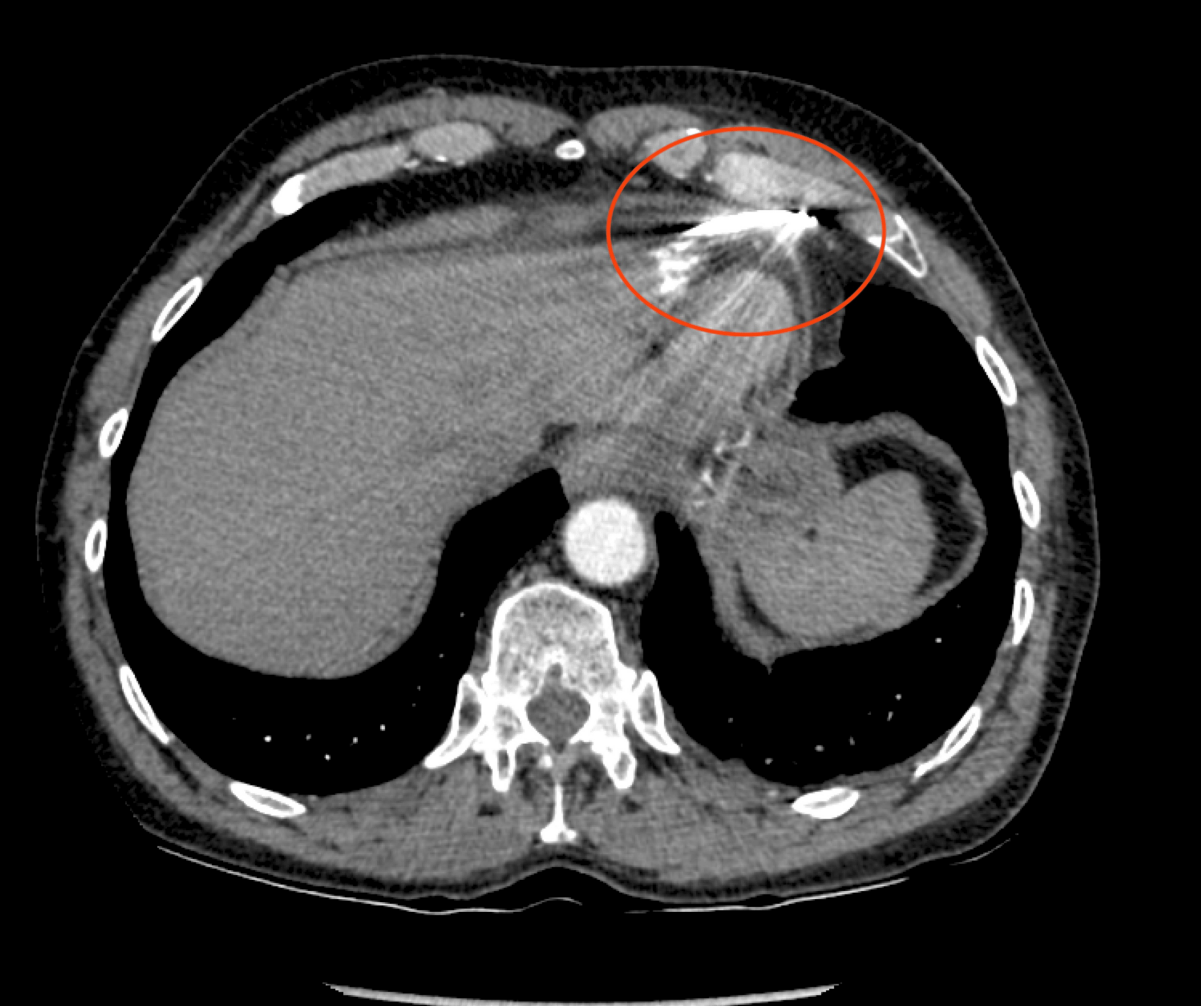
Pacemaker Lead Perforating Right Ventricle (RV) Found on Image Review 2-D computed tomography demonstrating RV lead perforation with the distal lead tip (red circle) within the anterior chest wall.
